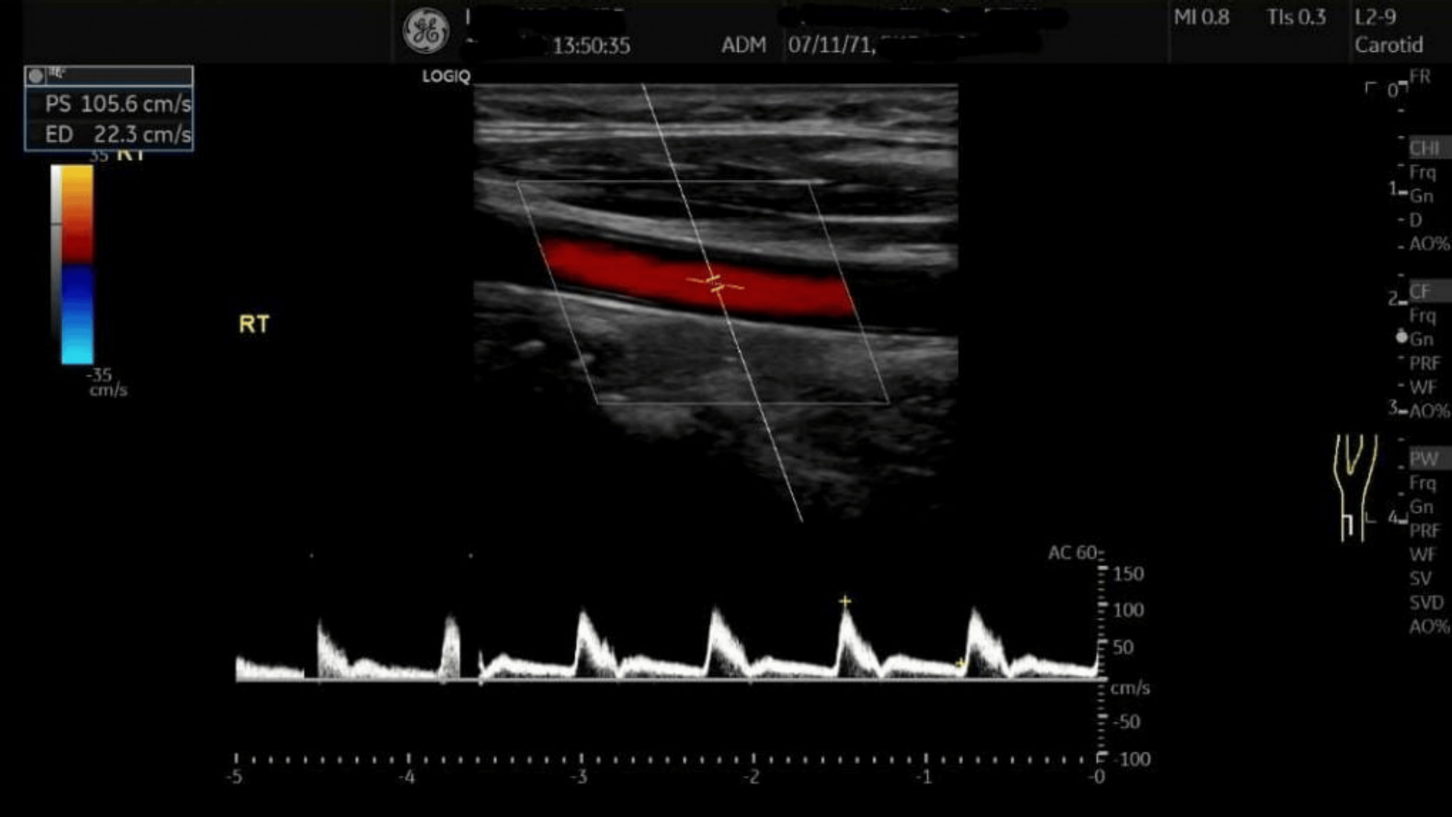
Doppler ultrasound of the carotids indicates normal findings
